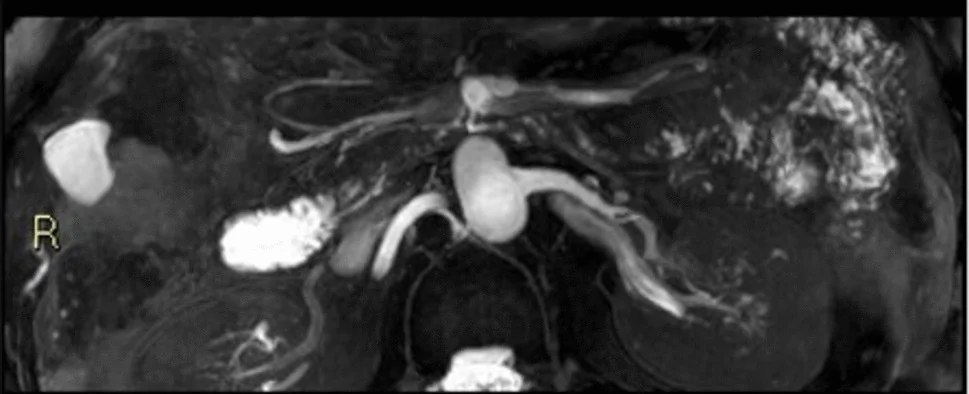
Perivascular cuffing of the left renal artery, extending from its origin to the hilum along the main lobar and interlobar branches, with mild hypointensity on T2-weighted images, and no significant diffusion restriction on DWI sequences
